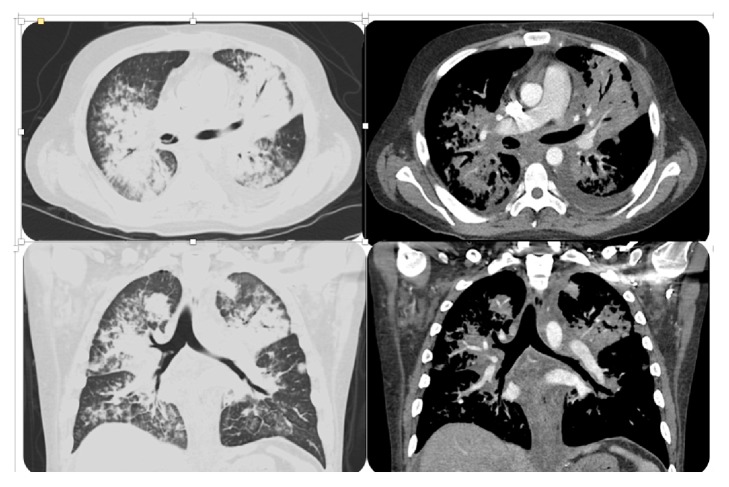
Computed tomography of the chest showed diffuse peribronchovascular consolidative opacities with surrounding ground glass opacities, interlobular septal thickening, and infiltrative soft tissue densities throughout the mediastinum. There is also a linear filling defect in the bronchus intermedius.
